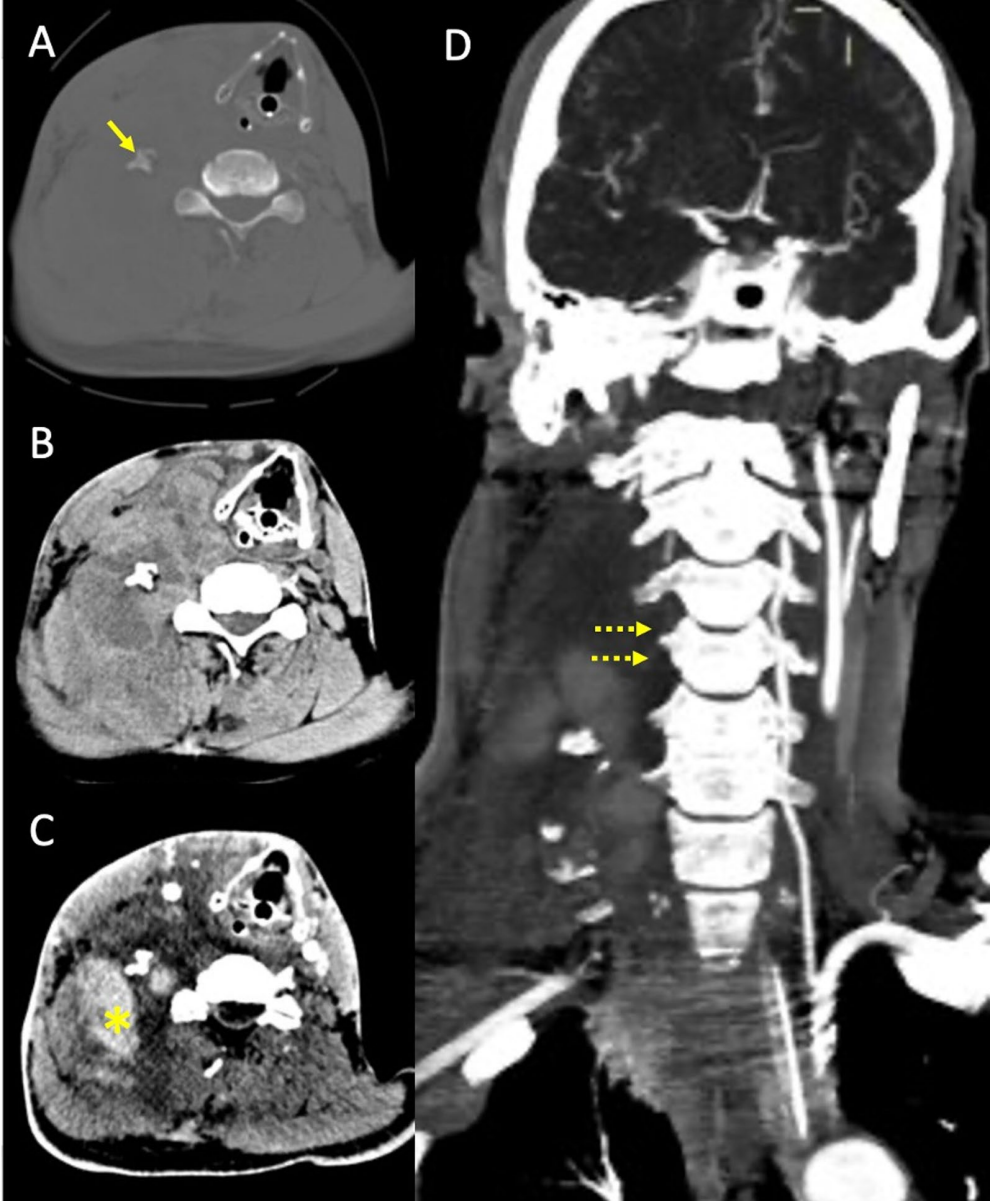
Right vertebral artery rupture after motor vehicle collision in a patient in the seventh decade of life. Non-enhanced CT scan (A and B) at the level of C5 shows fracture and lateral dislocation of the right transverse process (arrow), with ipsilateral distinct collection in the soft tissues of the neck (B). CTA (C) shows contrast extravasation in the right lateral cervical soft tissues, consistent with acute hematoma (asterisk), with lack of opacification of the right vertebral artery, best seen in the maximum intensity projection reconstruction (D, dotted arrows), most consistent with vertebral artery avulsion
